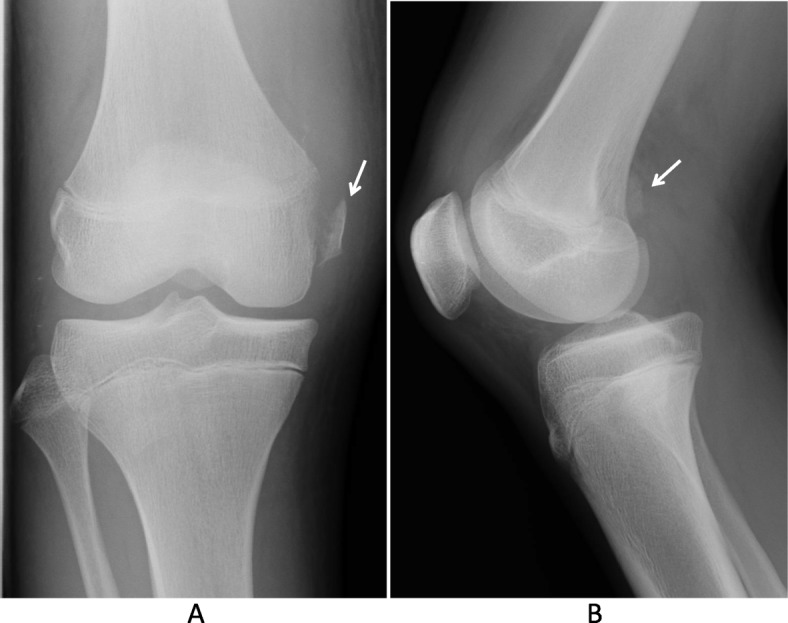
a and b Plain radiographs of the injured knee. Anteroposterior (a) and lateral (b) radiographs showing an avulsion fracture of the medial collateral ligament (*arrow*, a) and a bone fragment in the popliteal space (*arrow*, b)
